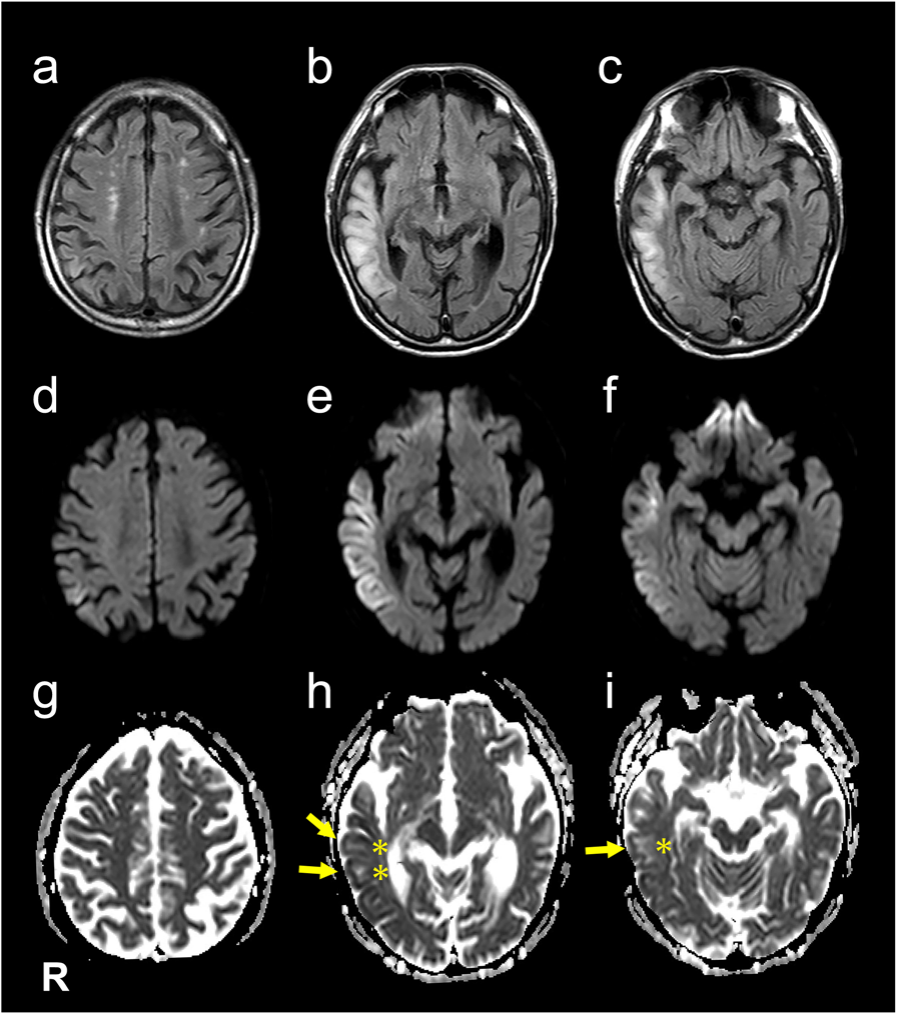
MR imaging findings. Fluid attenuated inversion recovery (FLAIR), (a-c), diffusion weighted imaging (DWI), (d-f), and apparent diffusion coefficient (ADC), (g-i) images of the case at first presentation. FLAIR revealed high-intensity lesions in cortical and subcortical areas of right parieto-temporal lobes. Cortical areas were hyperintense on DWI and hypointense on ADC (arrows). Subcortical areas were hyperintense on DWI and hyperintense on ADC indicating vasogenic edema (asterisks)
